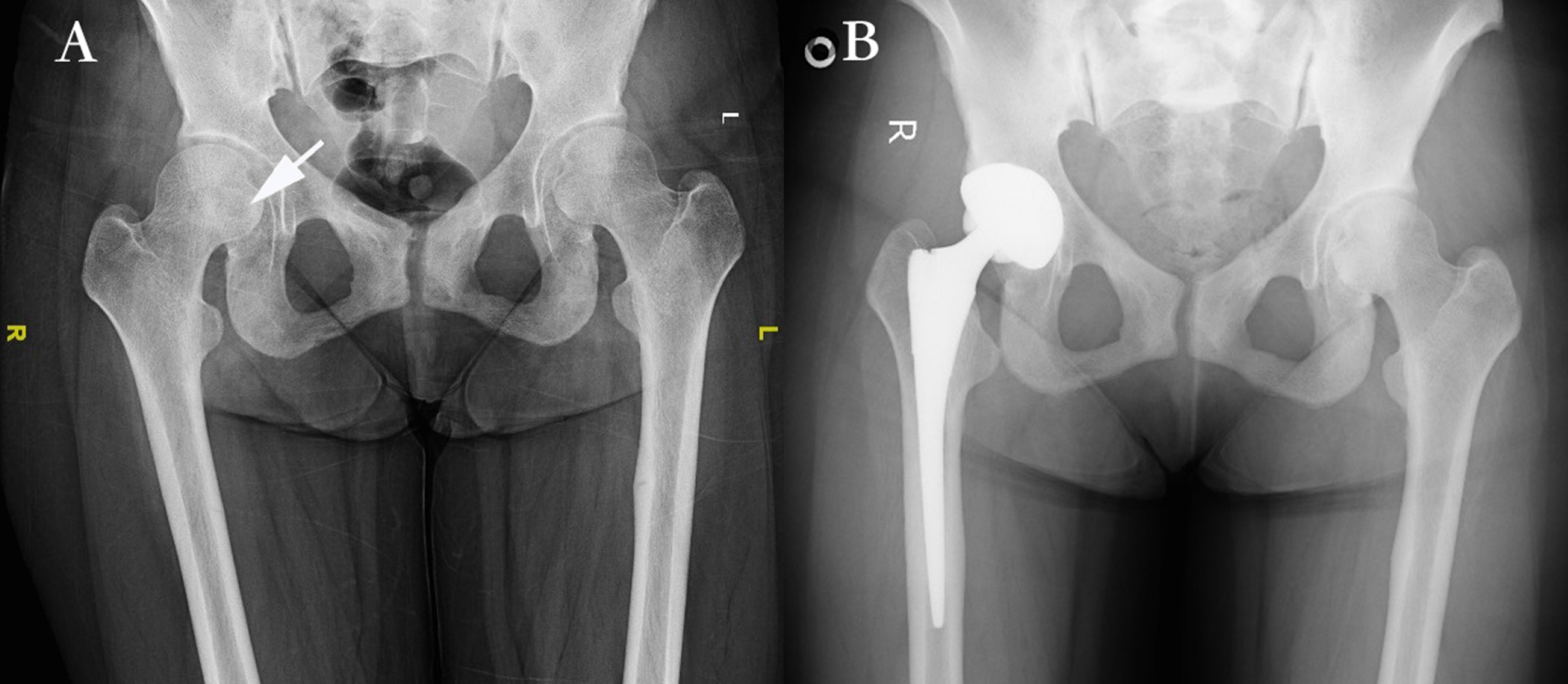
X-ray examination before and after joint arthroplasty in the treatment of tumor-induced osteomalacia patients. 2A: Osteoporosis and the causative lesion in a 52-year-old male TIO patient are shown on X-ray before joint arthroplasty. 2B: The patient underwent joint arthroplasty after one year. Osteoporosis was significantly improved, as shown by X-ray.
